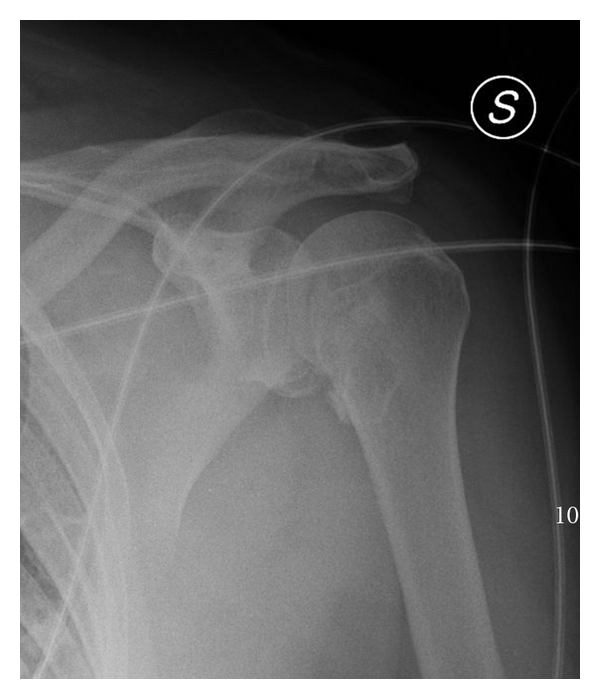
X-ray shows fracture of the humeral head and intrarotation of the arm.
